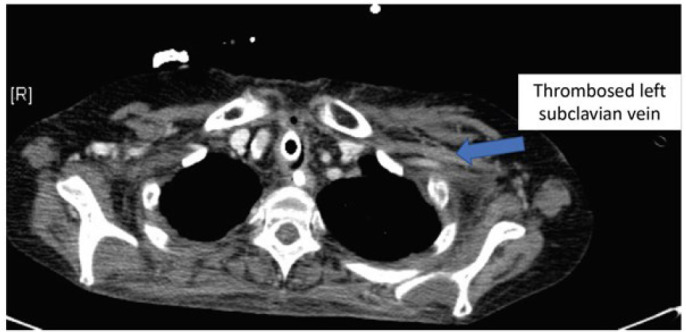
Computed tomography angiography showing thrombosed left subclavian
